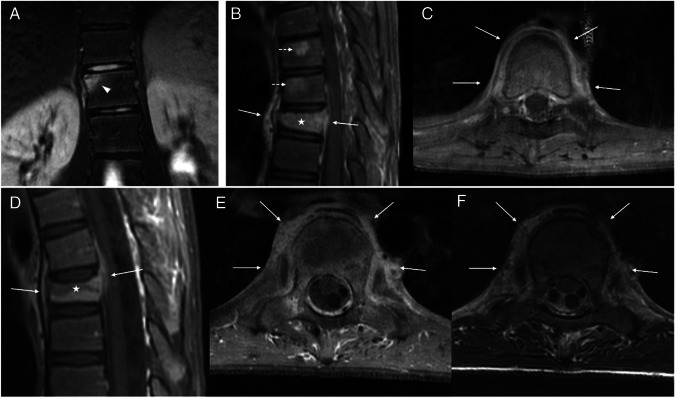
Types of inflammatory changes in vertebral lesions: magnetic resonance images obtained from a 16-year-old girl (a), a 12-year-old boy (b, c), and a 14-year-old boy (d–f). a Coronal T2-weighted fat-saturated image of the dorsolumbar spine shows a lesion of the body of dorsal (D) vertebra 12 with only bone edema (*arrowhead*). b, c Sagittal (b) and axial (c) T1-weighted contrast-enhanced fat-saturated images of the dorsal spine reveal vertebral height loss and contrast enhancement of the body of D8 (*asterisk*), and the presence of enhancing soft tissue inflammation with a peri-vertebral distribution (*arrows*). Note also the enhancing inflammatory lesions of the D6 and D7 vertebral bodies (*broken arrows*). d–f Sagittal (d) and axial (e) T1-weighted contrast-enhanced fat-saturated images and axial T2-weighted (f) image of the dorsal spine show platyspondyly of D6 (*asterisk*) and extensive peri-vertebral soft tissue inflammation (*arrows*). Note the involvement of the costovertebral joints
